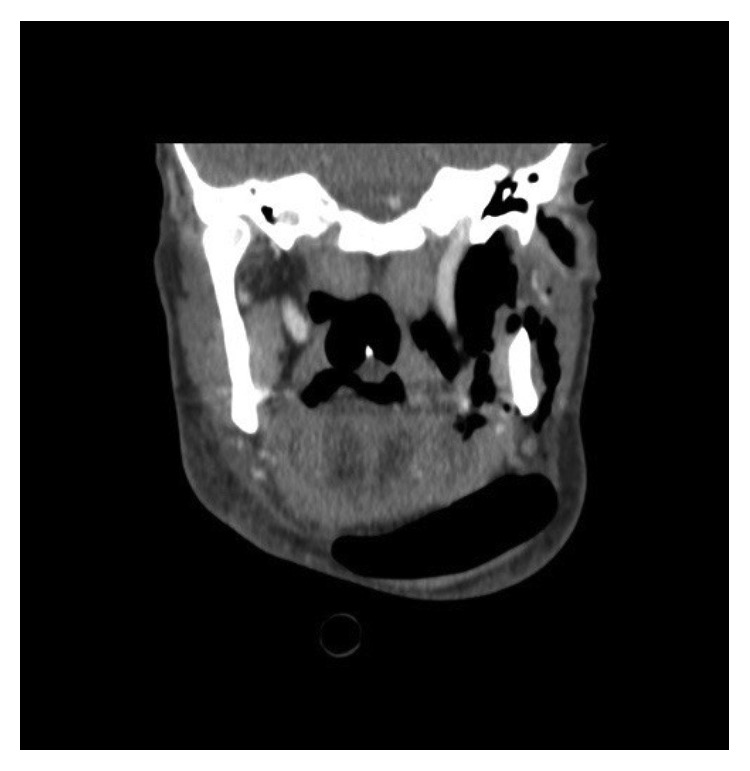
Coronal reformats of the axial CT of the skull base and neck demonstrating extensive subcutaneous emphysema within the left submandibular, submental, parotid, and parapharyngeal spaces.
